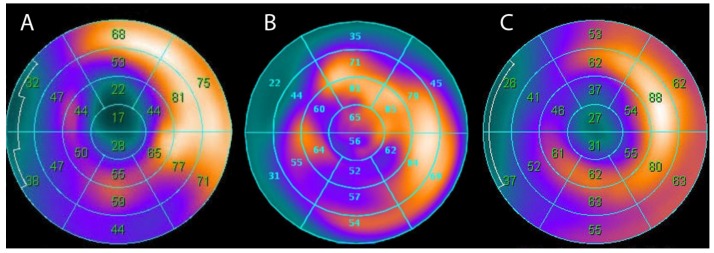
Clinical example of “flow-metabolism mismatch.” 76 years old male with a clinical history of anterior myocardial infarction was admitted to the hospital due to unstable angina. Coronary angiography revealed triple coronary artery disease. Myocardial perfusion imaging (MPI) (99mTc-MIBI SPECT at rest) (A) demonstrated perfusion defect in apex and apical septal, anterior and lateral segments, while myocardial viability test (FDG-PET) (B) in the same segments revealed viable myocardium. Surgical revascularisation was performed. Six months after coronary artery bypass grafting (CABG) patient‘s functional class was increased, he had no angina and MPI (99mTc-MIBI SPECT at rest) (C) revealed slightly better perfusion in the same segments.
